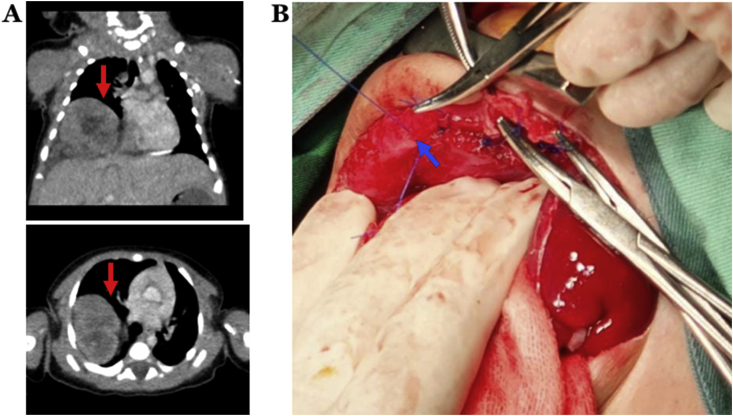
A) Computed tomography (CT) scanning indicated right diaphragmatic elevation and kidney inside the right hemithorax. B) Intraoperative findings displayed neither elevation of right diaphragmatic dome nor any other organ displacement.
